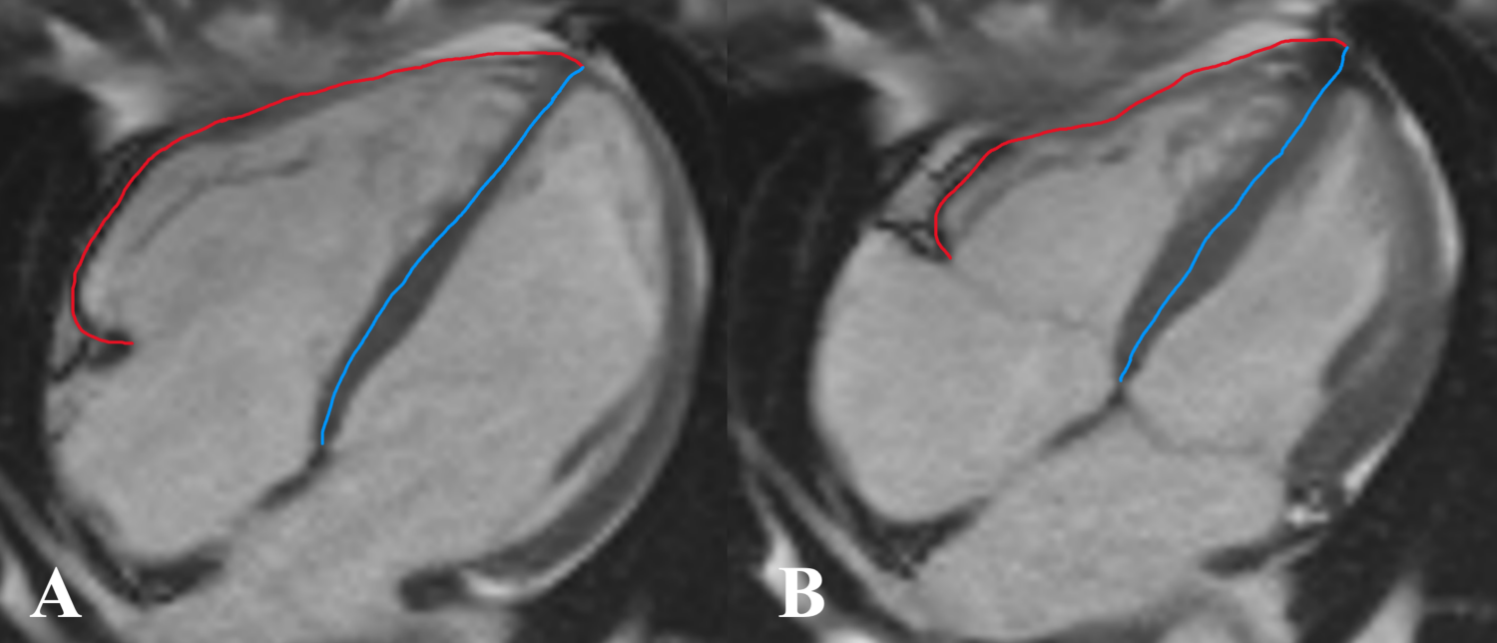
Measurement of right ventricular end-diastolic remodeling index (RVEDRI) (A) and right ventricular end-systolic remodeling index (RVESRI) (B) on the four-chamber cine image of cardiac magnetic resonance imaging (CMR). RVEDRI and RVESRI were the ratios of lateral free wall length (red line) to interventricular septal height (blue line).
